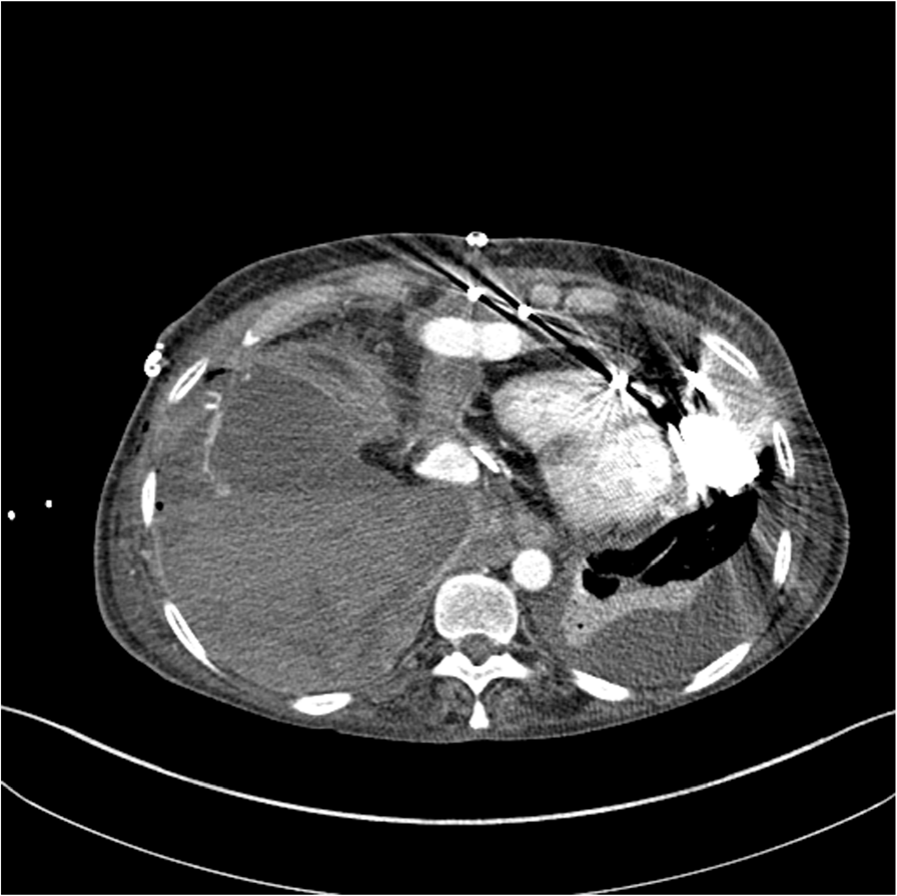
Axial CT-angiography image shows active arterial bleeding in the thorax from the IX intercostal right artery
